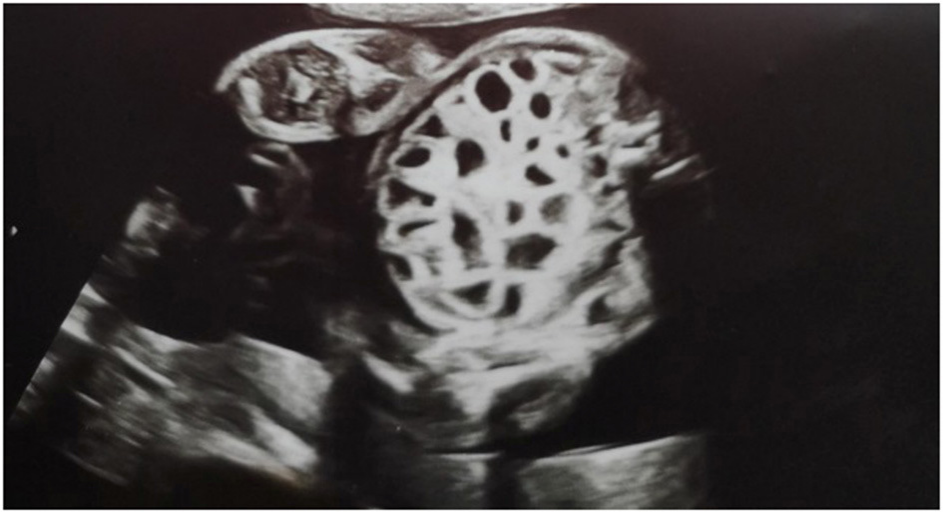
Ultrasound views at 24 weeks and 4 days of gestation showing multiple dilatation of the bowel loops filled with fluid.
